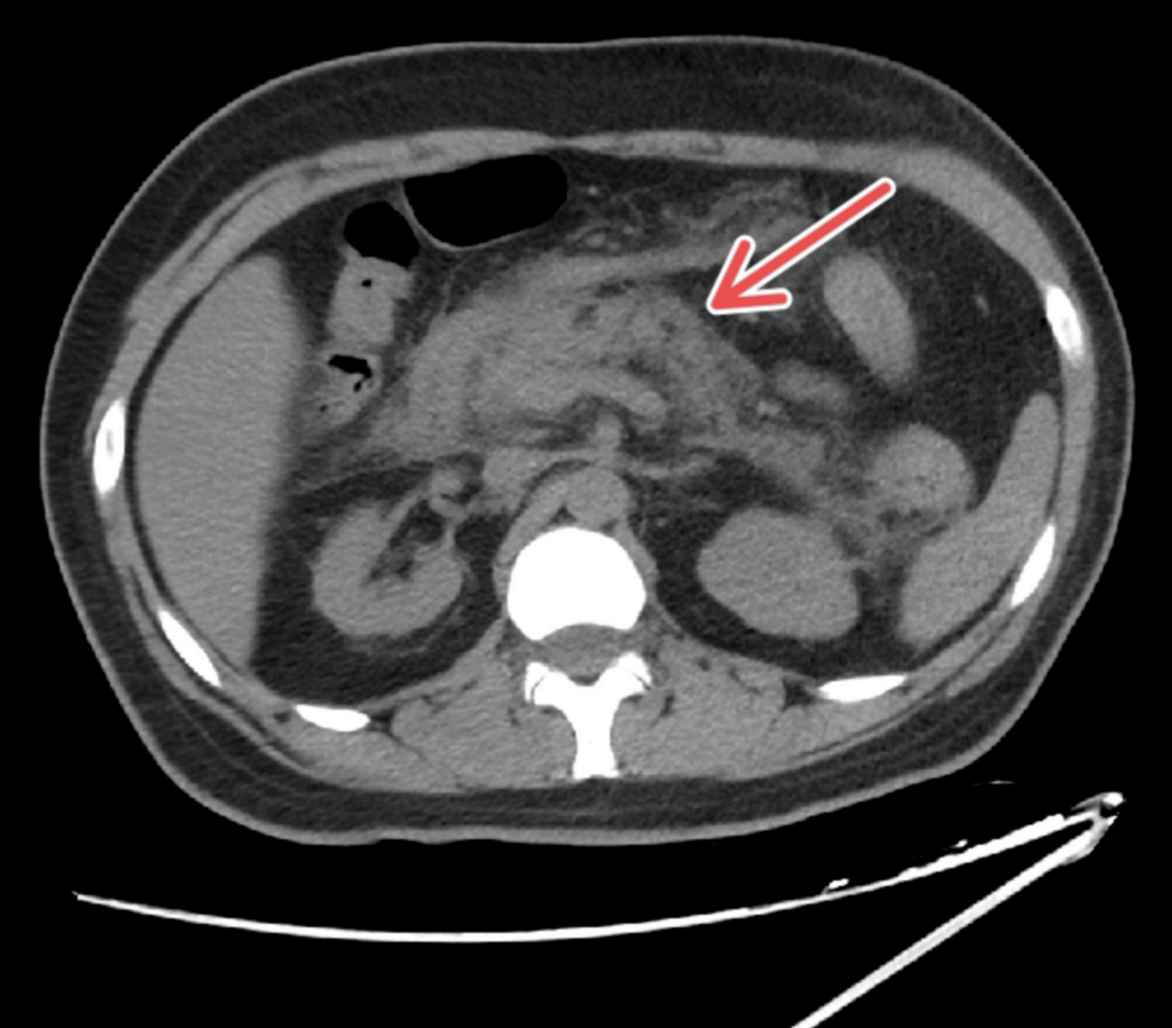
CT of the Abdomen and Pelvis Without Contrast Abdominal CT at the level of the epigastrium. The red arrow marks the peri-pancreatic stranding, compatible with the diagnosis of acute pancreatitis.
